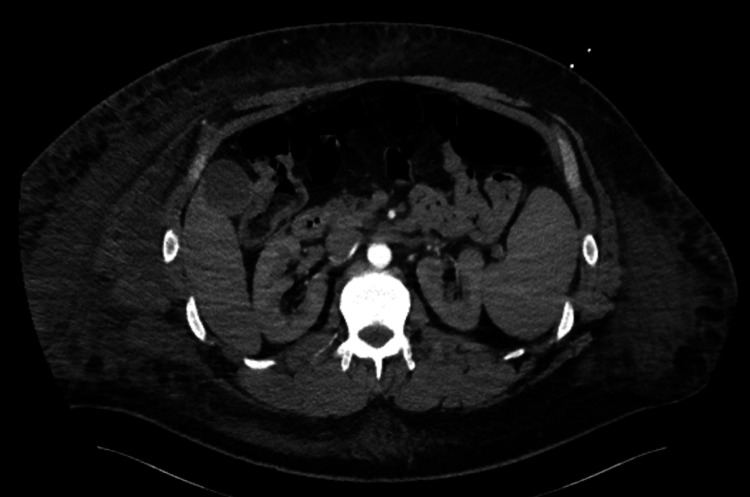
CT of the abdomen demonstrating subcutaneous edema with air pockets throughout the right lower quadrant abdominal wall.
